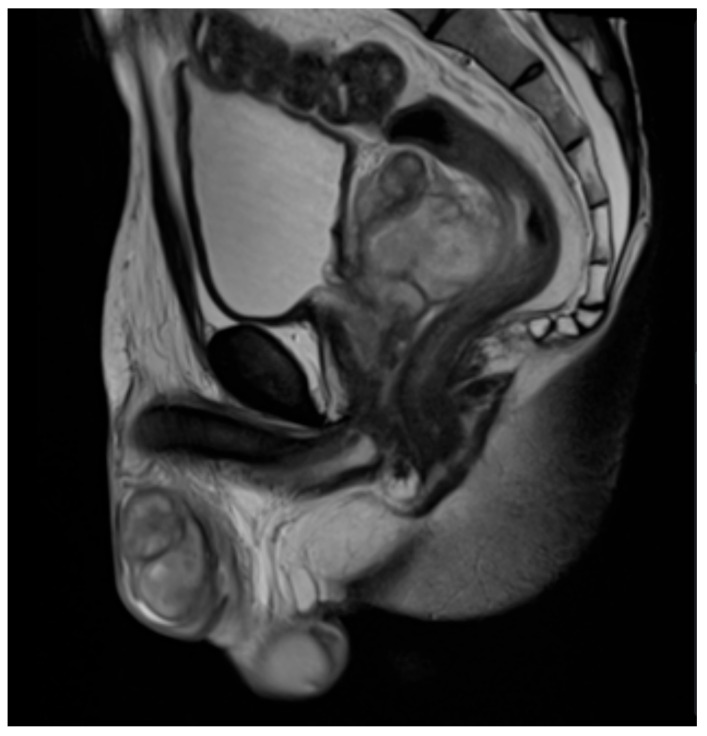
T2-weighted MRI displaying fluid collections in the right testicle, epididymis, and ductus deferens. Inflammation extends to the seminal vesicles and prostate, suggesting abscess formation characteristically seen in seminal vesicle and prostate involvement of TB. Heterogeneous contrast enhancement is also visualized. Case courtesy of Lam Van Le, Radiopaedia.org, rID: 206329, licensed under CC-NC-BY-SA 3.0 [71].
